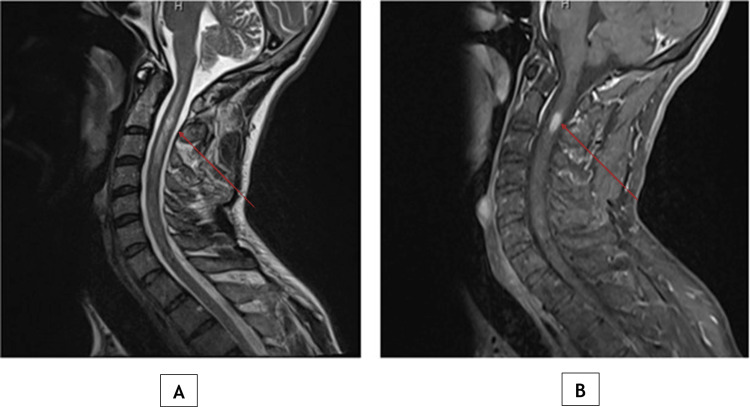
Cervical spine MRI showing an enhancing lesion at C2 to C3 consistent with demyelination Sagittal T2-weighted MRI (A) shows the arrow indicating a focal intramedullary hyperintense lesion in the cervical cord at C2-C3. Post-contrast sagittal T1-weighted MRI (B) shows the arrow indicating corresponding enhancement of the same lesion, consistent with active inflammatory demyelination. In conjunction with the intracranial lesion, these findings support the diagnosis of tumefactive multiple sclerosis.
